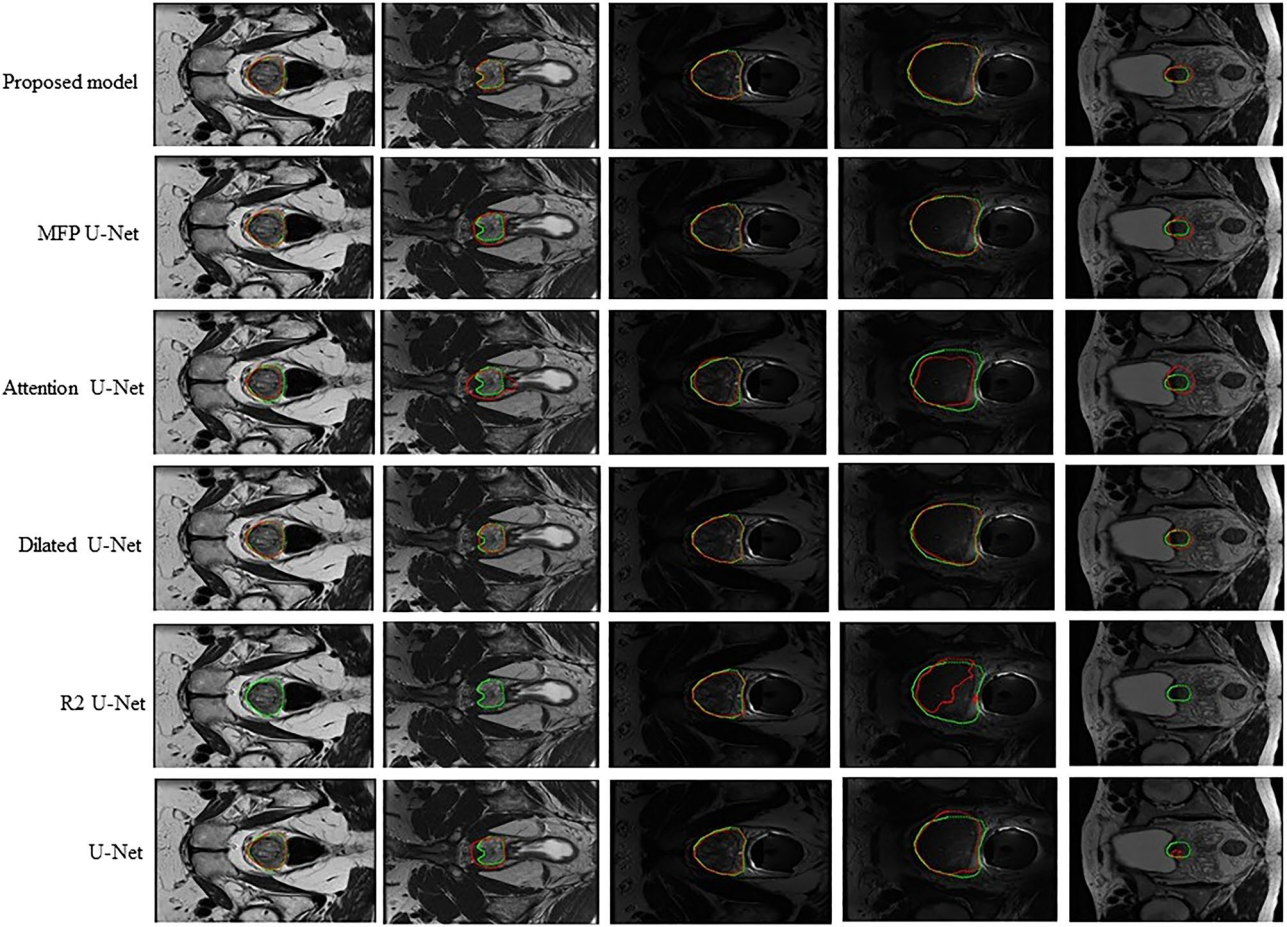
Representative results of segmentation achieved by the proposed model in comparison with other U-Net based models on multi-state MRI dataset.
